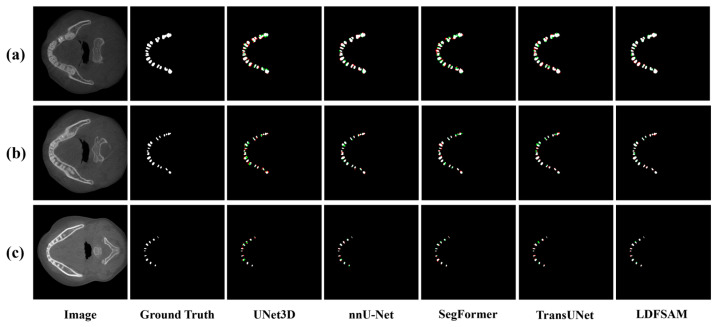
Visual comparison on 3D CBCT Tooth datasets. (a) Representative case 1; (b) Representative case 2; (c) Representative case 3. White, green and red indicate correct segmentation, under-segmentation and over-segmentation, respectively.
